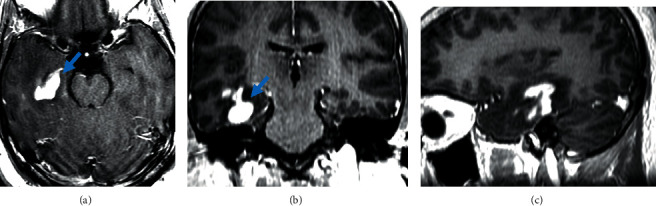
Contrast-enhanced axial (a), coronal (b), and sagittal (c) views of MRI brain showing CNS relapse. Images reveal homogenous enhancement (blue arrows) in periventricular tissues of right temporal lobe.
